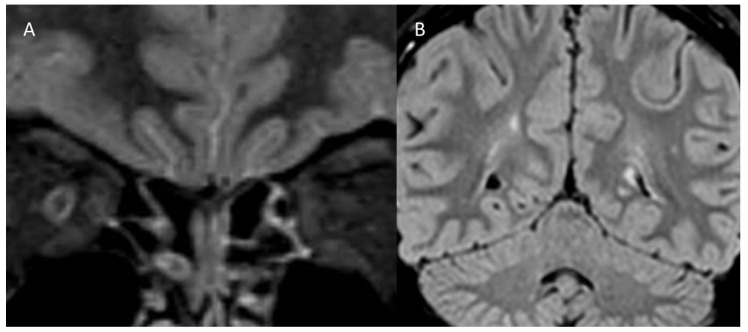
Magnetic resonance imaging scans of case 3. (A) Coronal short inversion time recovery sequence shows hyperintensity of the right optic nerve. (B) Coronal fluid-attenuated inversion recovery section of the brain demonstrates multiple bilateral hyperintense periventricular and juxtacortical white matter lesions.
